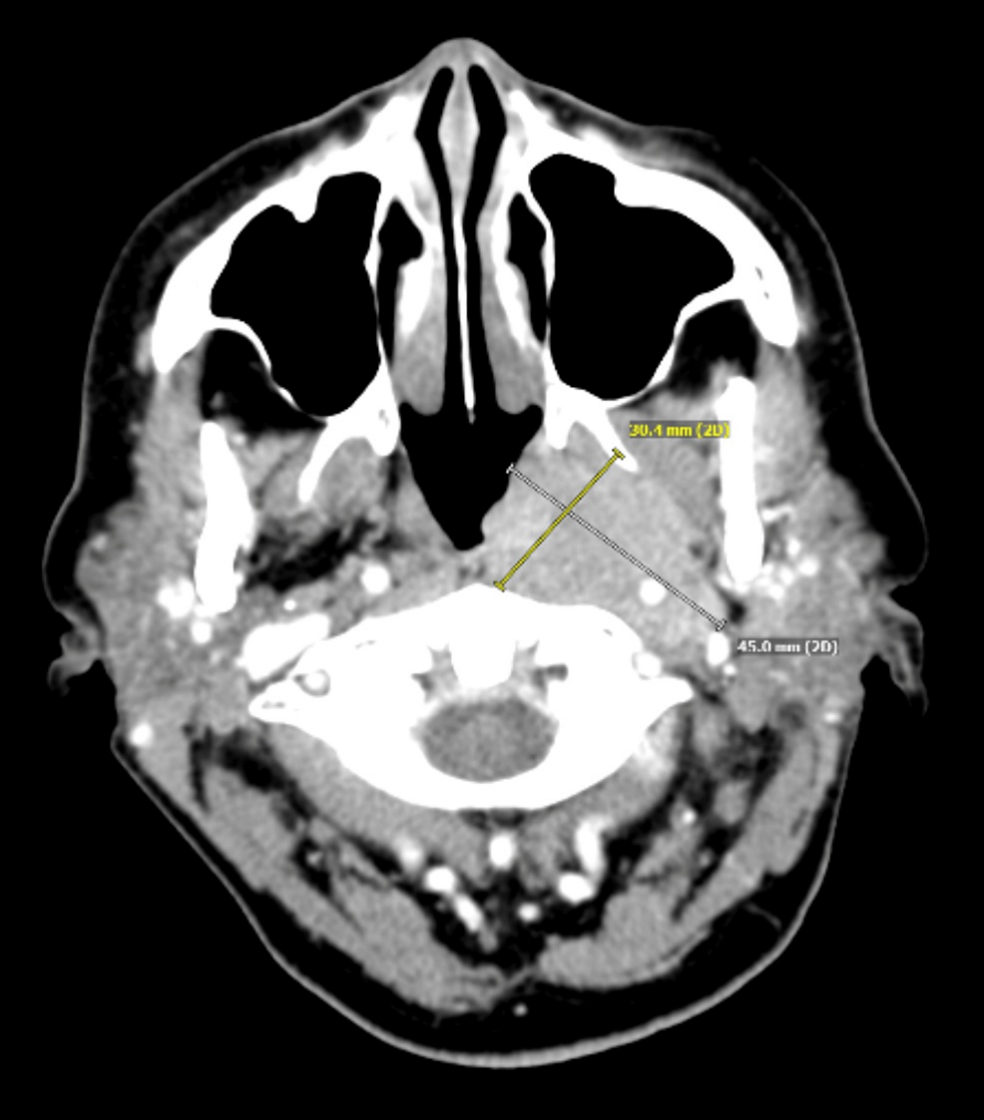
Computed tomography of the neck (axial view) demonstrating an ill-defined trans-spatial mass in the left upper neck below the base of the skull.
